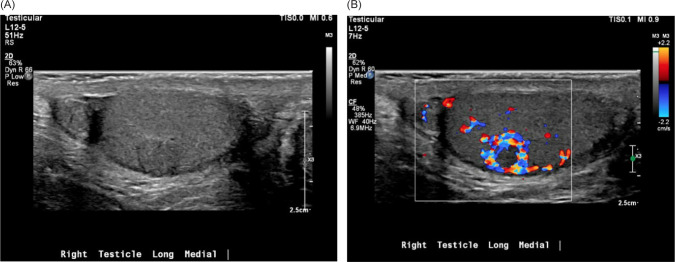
(A) Scrotal ultrasound demonstrating long view of the medial right testicle with echogenic, heterogeneous, and well-circumscribed mass. (B) Doppler ultrasound demonstrating hypervascularity of the previously characterized mass.
